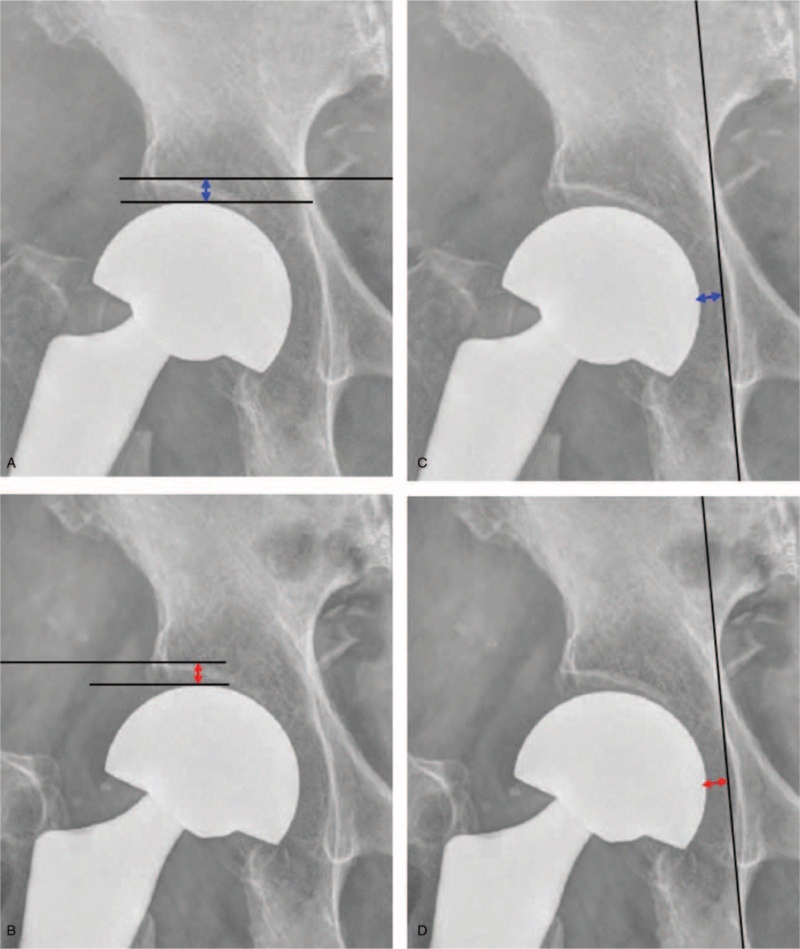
Measuring the amount of acetabular erosion. (a) Immediate postoperative radiograph measuring the distance from acetabular line to the level of prosthesis (A). (b) Follow-up radiograph measuring the same distance (B). (c) Immediate postoperative radiograph measuring the distance from Kohler line to prosthesis (C). (d) Follow-up radiograph measuring the same distance. Superior acetabular erosion calculated as (A and B) in mm. Medial acetabular erosion calculated as (C and D) in mm.
